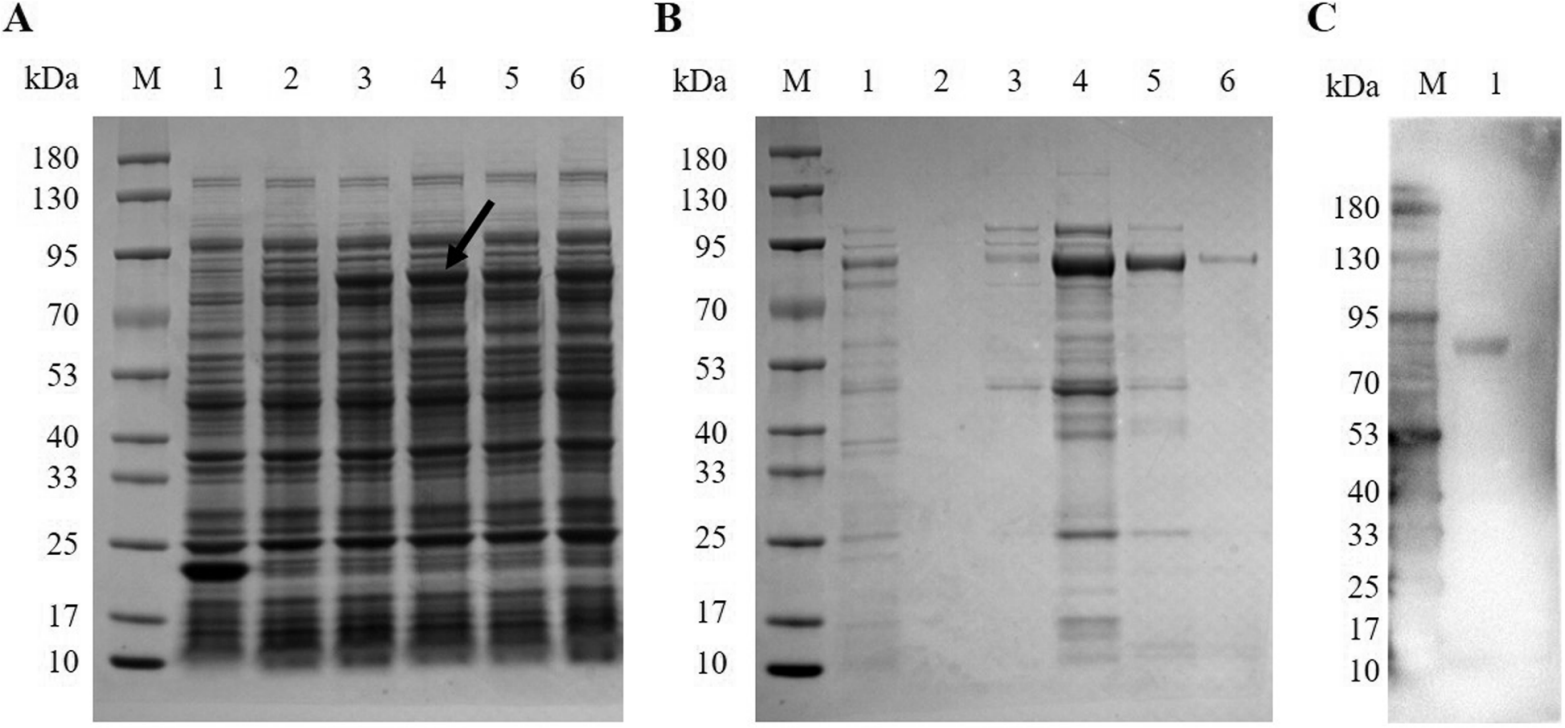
Expression and analysis of recombinant Apical membrane antigen 1 (rAMA1) of *Toxoplasma gondii*. A Sodium dodecyl sulfate-poly-acrylamide gel electrophoresis (SDS-PAGE) analysis of rAMA1 incubated at different times. M: Marker; Lane 1: Induced control culture of cells with empty vector pET-32a ( +); lane 2–6: Expression of rAMA1 after 0, 2, 4, and 6 h of induction separately at 37 ℃. The arrowhead indicates that the highest expression level of rAMA1 was 4 h after induction at 37 ℃. B SDS-PAGE analysis of rAMA1 eluted with imidazole at different concentrations. M: Marker; Lane 1: Control culture of unpurified cell lysate after ultrasound; lane 2–6 Elution of rAMA1 with imidazole at 50, 100, 200, 400, 500 mM. C Western blot (WB) analysis of rAMA1 with His-tag. M: Marker; lane 1: Mixed multiple 400 mM imidazole-eluted rAMA1
